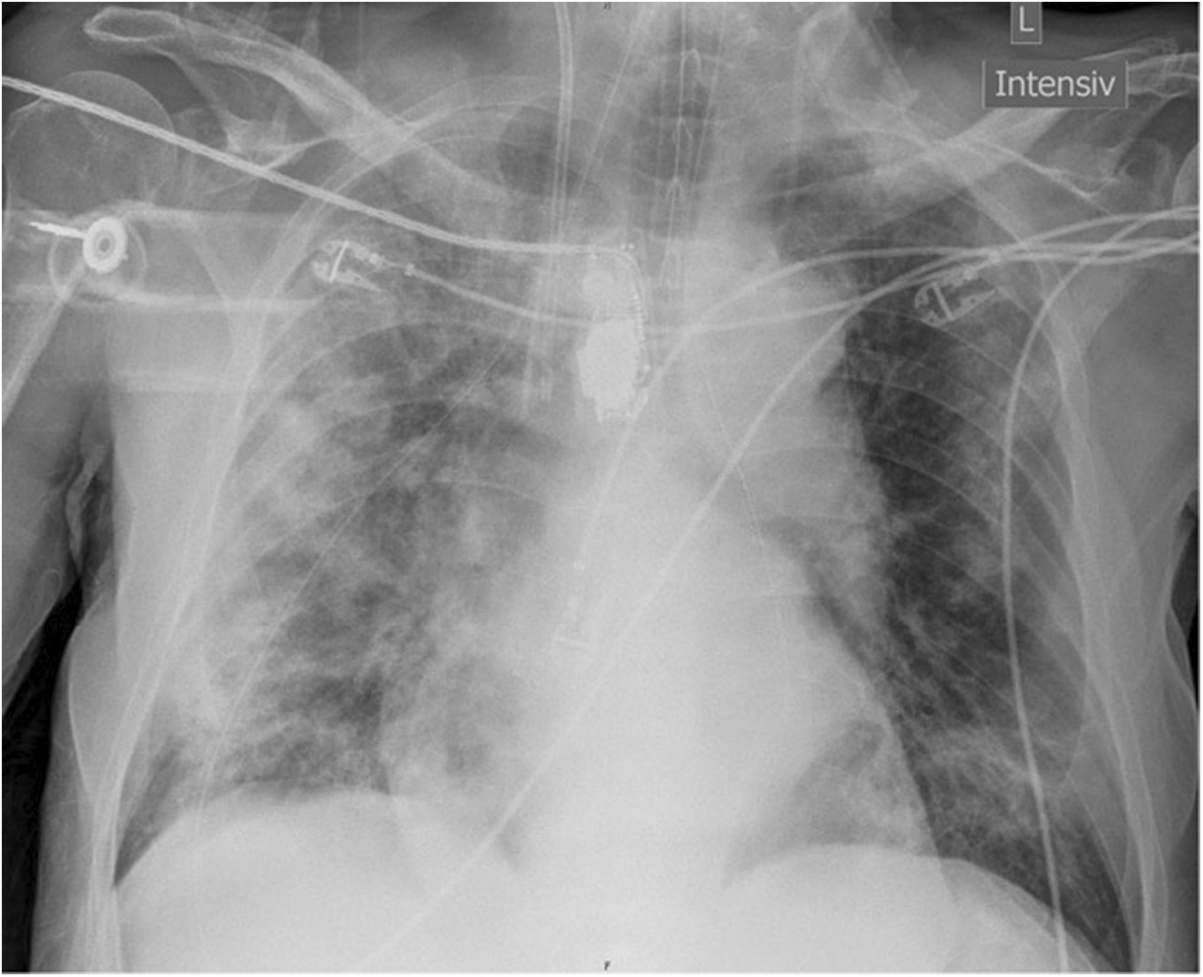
Chest radiography showed Covid‐19 typical bipulmonary infiltrates, which already occupied 70% of the patient's lung parenchyma. Chest radiography showed bipulmonary infiltrates typical of Covid‐19, which affected 70% of the lung parenchyma of the 90‐year‐old patient already on the day of admission to the ICU (day 2).
